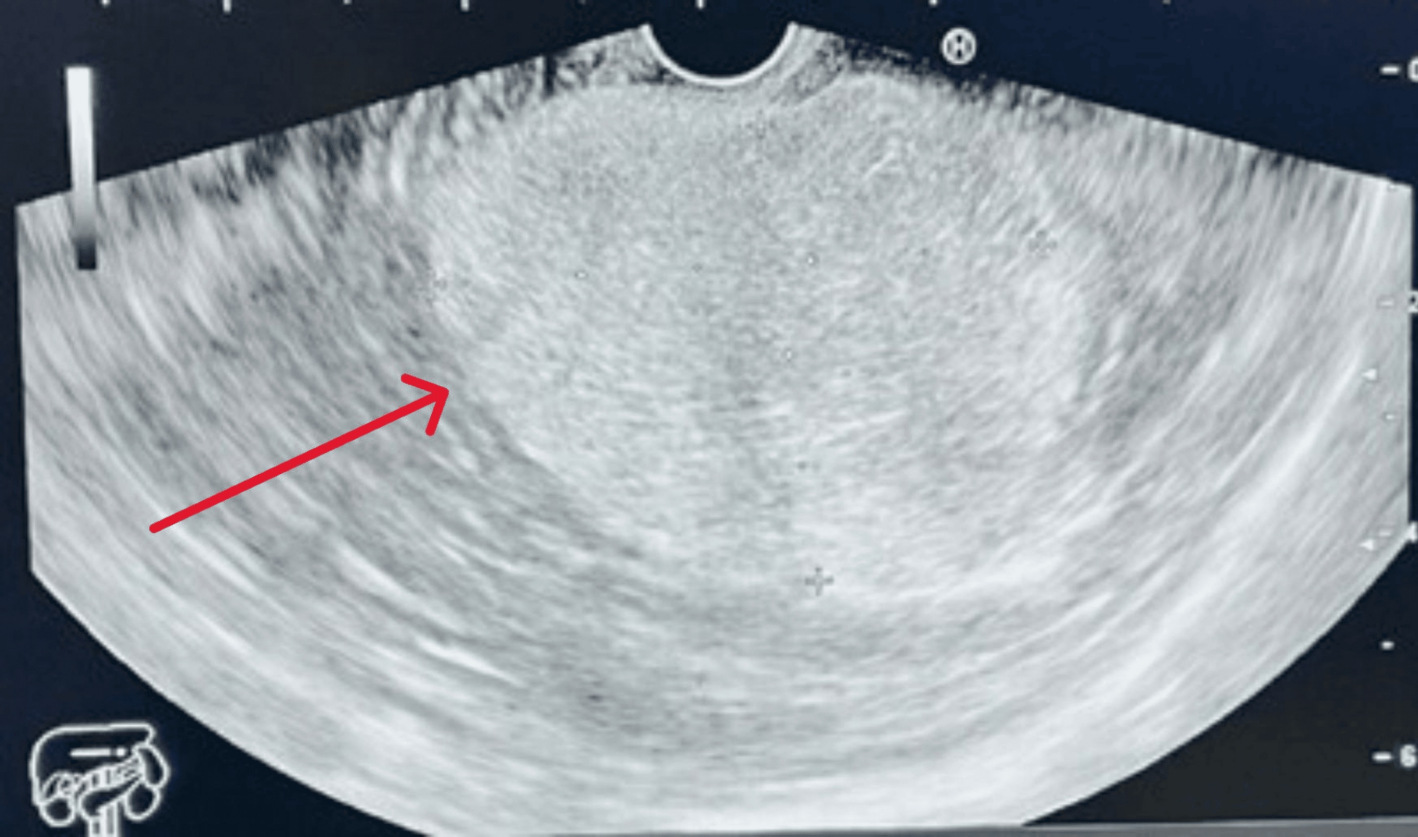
EUS, A 53 X 39 mm well defined, homogenous, hyperechoic subepithelial lesion EUS: endoscopic ultrasound EUS showing a well-defined hyperechoic mass, measuring 53 x 39 mm (red arrow)
